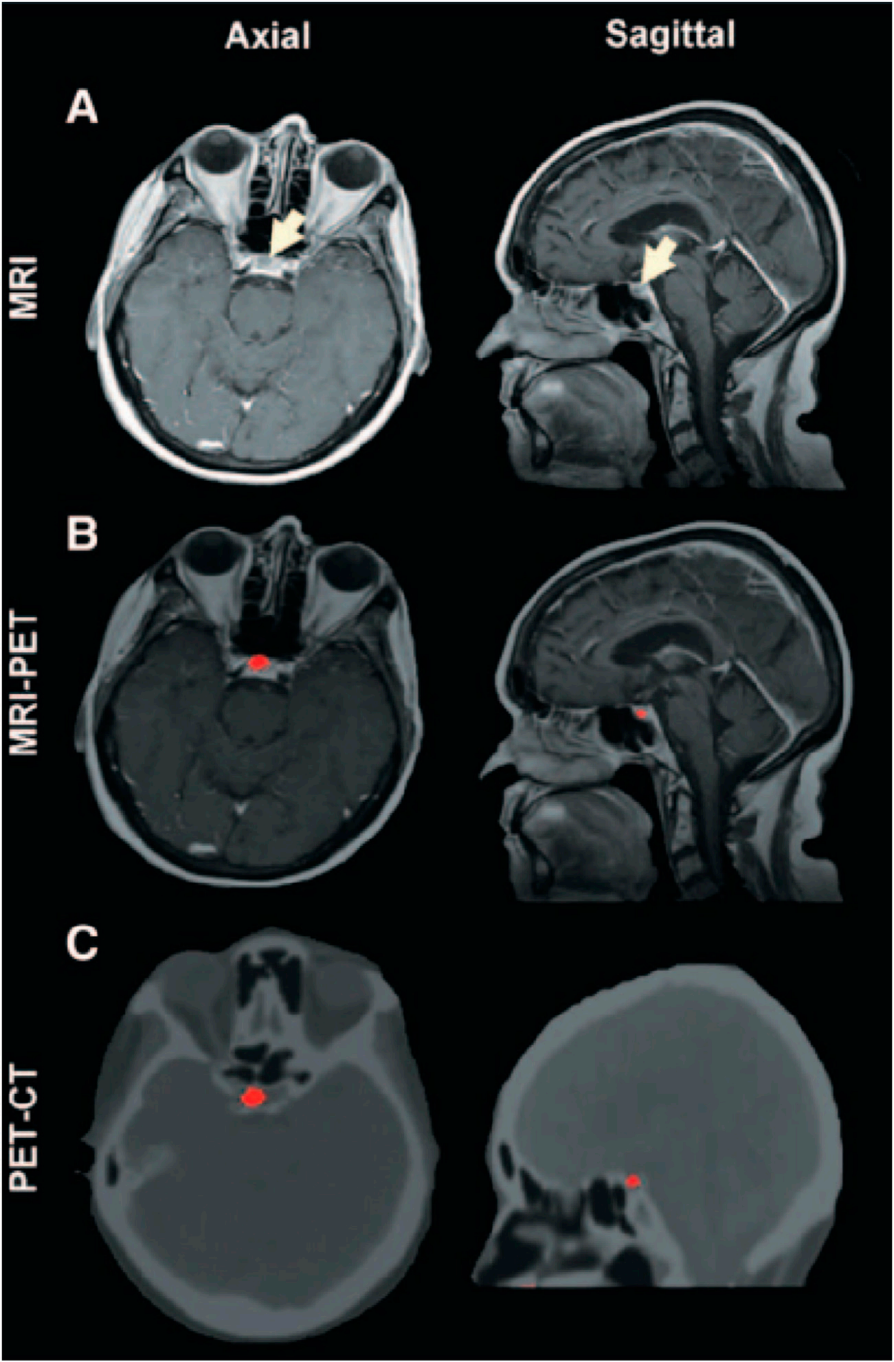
Multimodal Imaging of Particle Uptake in a Pituitary Lesion. (A) Multiplanar contrast-enhanced MR axial and sagittal images of patient, taken 72 h post-injection, demonstrate a subcentimeter cystic focus (arrows) within the right aspect of the anterior pituitary gland. (B) Co-registered axial and sagittal MRI-PET images show increased focal activity (red, 124I-cRGDY–PEG–C dots) localized to the lesion site. (C) Axial and sagittal PET-CT images further localize this activity to the right aspect of the sella. Reproduction with the permission of reference (Phillips et al., 2014). Copyright ^©^2014 American Association for the Advancement of Science.
